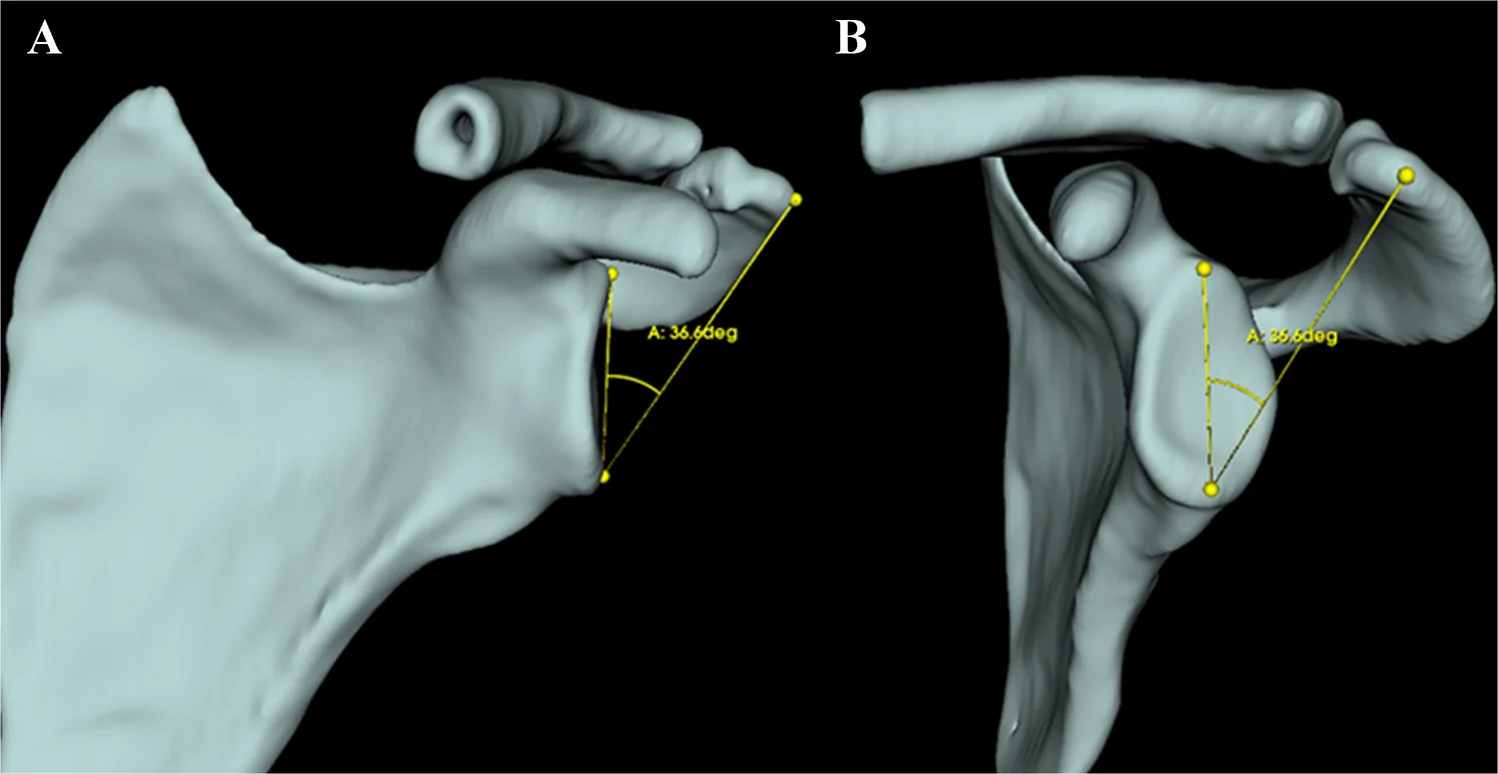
Demonstration of the measurement of critical shoulder angle (CSA) on three-dimensional computed tomography (3D-CT). A Anterior view of CSA measurement. B Lateral view of CSA measurement
